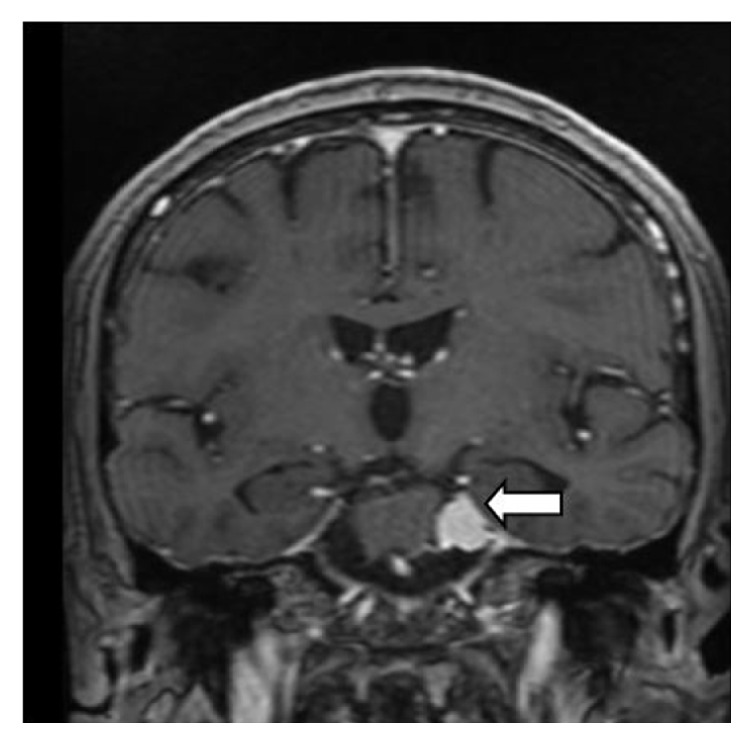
Cerebral MRI showing trigeminal meningioma: T1 coronal and sagittal sequence.
